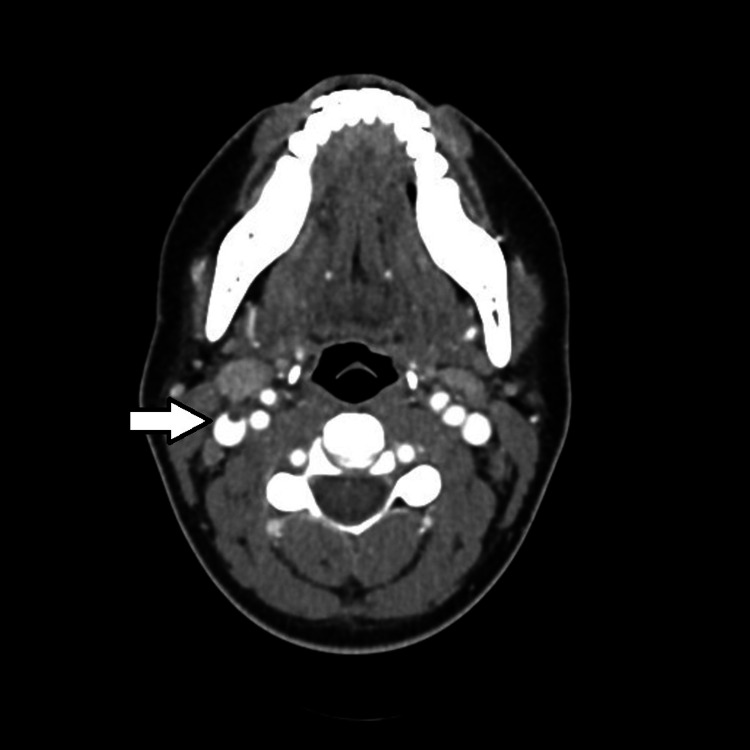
Initial CT with contrast demonstrating a filling defect in the right internal jugular vein.
